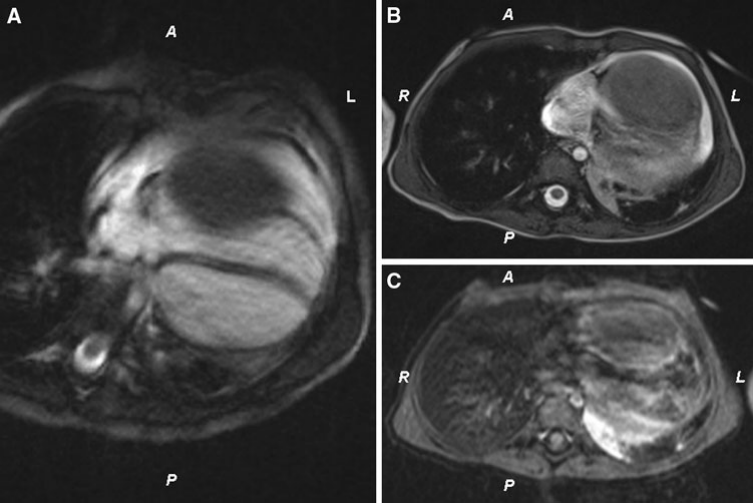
The presence of a solid tumor confirmed by magnetic resonance imaging
